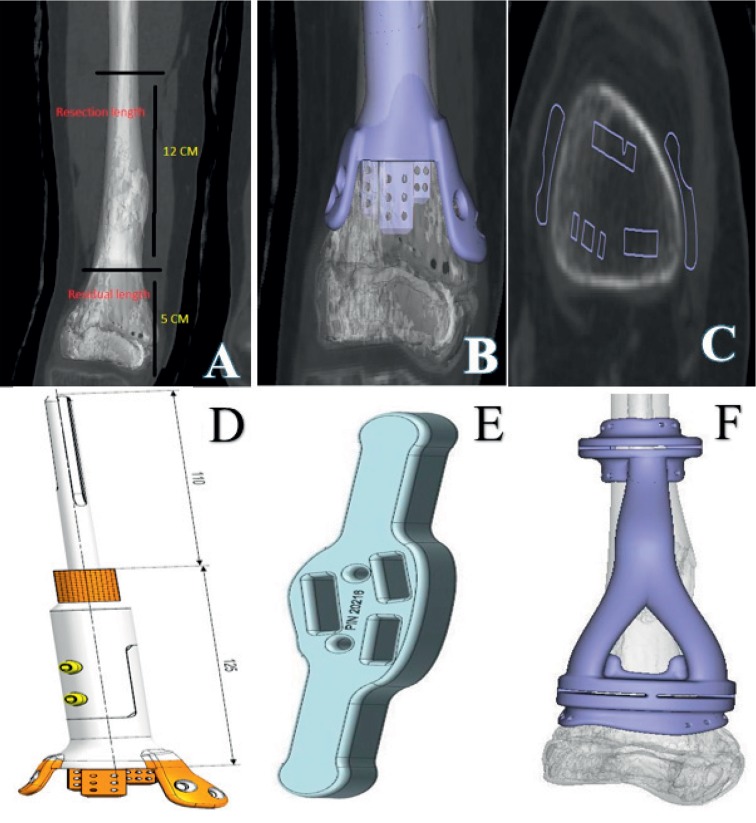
(a) A measuring CT scan of femur showing the resection length and residual metaphyseal-epiphyseal bone segment length. (b, c) Fitting of the implant design into the residual bone segment. (d) The final JSE design. (e) The 3D printed fin template which will match in size and dimensions to the metaphyseal surface of residual bone. (f) The 3D printed cutting guide which will be used to make distal and proximal bone osteotomies.
